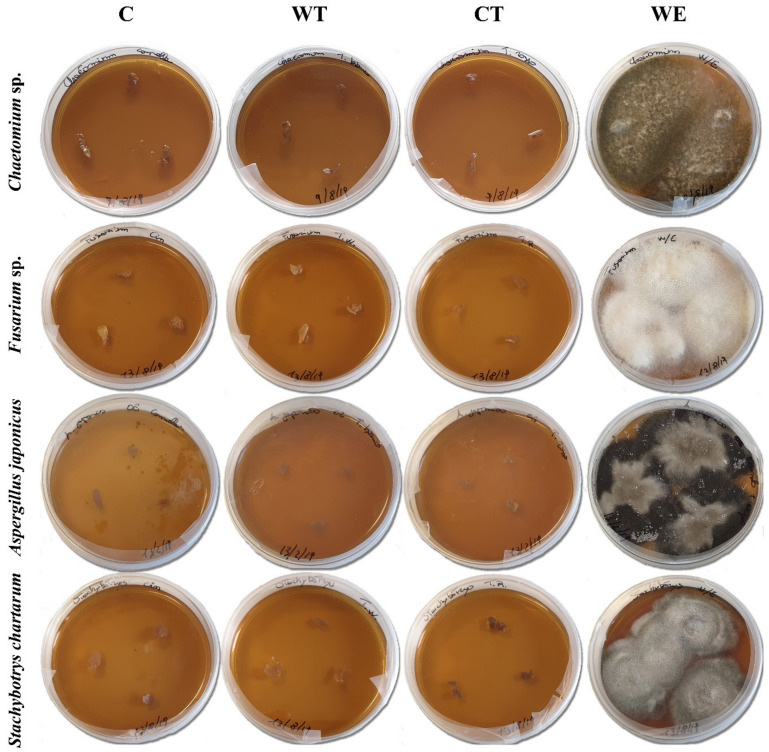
Results of cultural tests carried out on the fungal mycelia treated with the EOs MIC. (C: cinnamon; WT: wild thyme; CT: common thyme; WE: water-ethanol).
